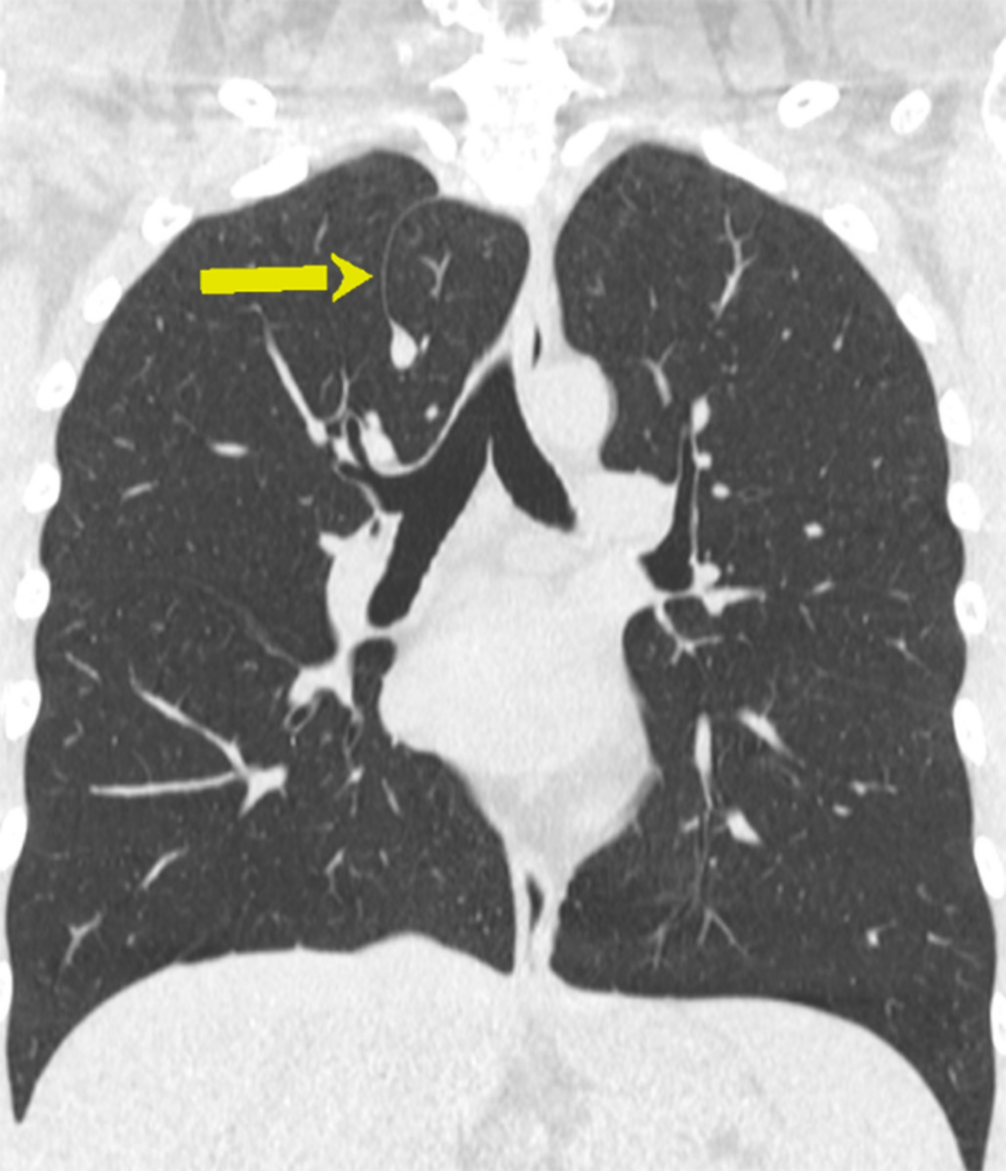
Computed tomography (CT) of the chest - coronal image; azygos lobe (arrow)
